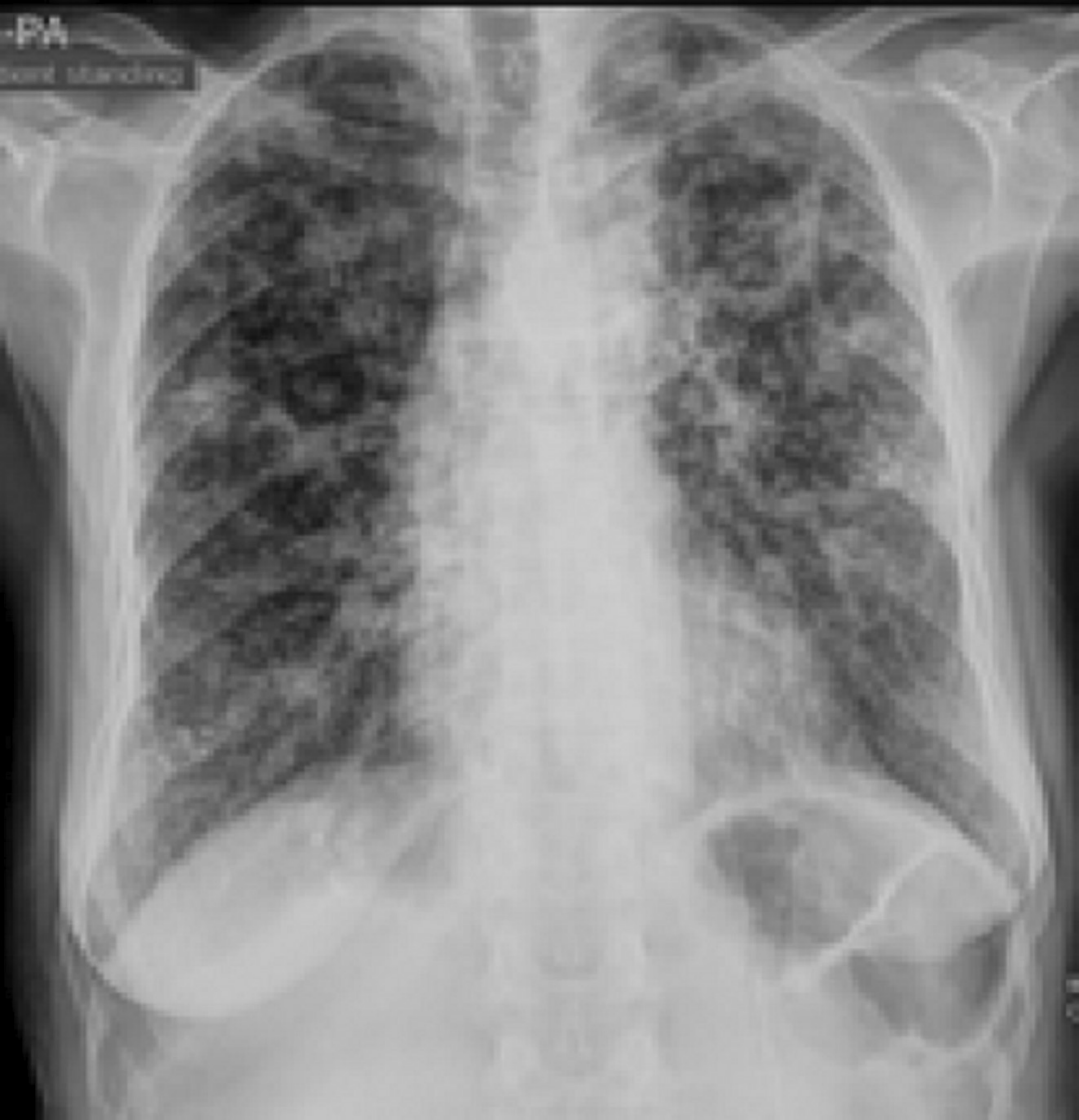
Chest radiograph, frontal projection (post treatment): The previously seen large opacity is resolved. Bilateral emphysematous changes are seen.
